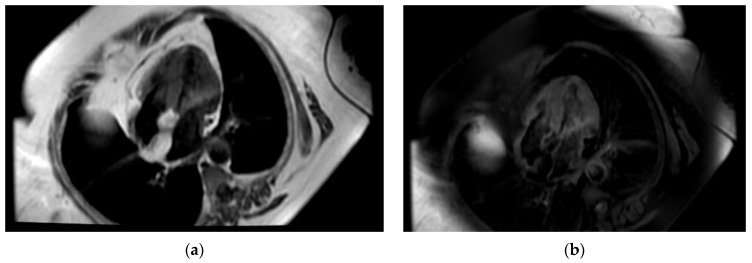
Cardiac MRI. (a) Double-inversion-recovery T1 sequence (4 chambers) revealing a hyperintense interatrial septum suggestive of fat; (b) double-inversion T1 sequence with fat suppression revealing a hypointense interatrial septum.
